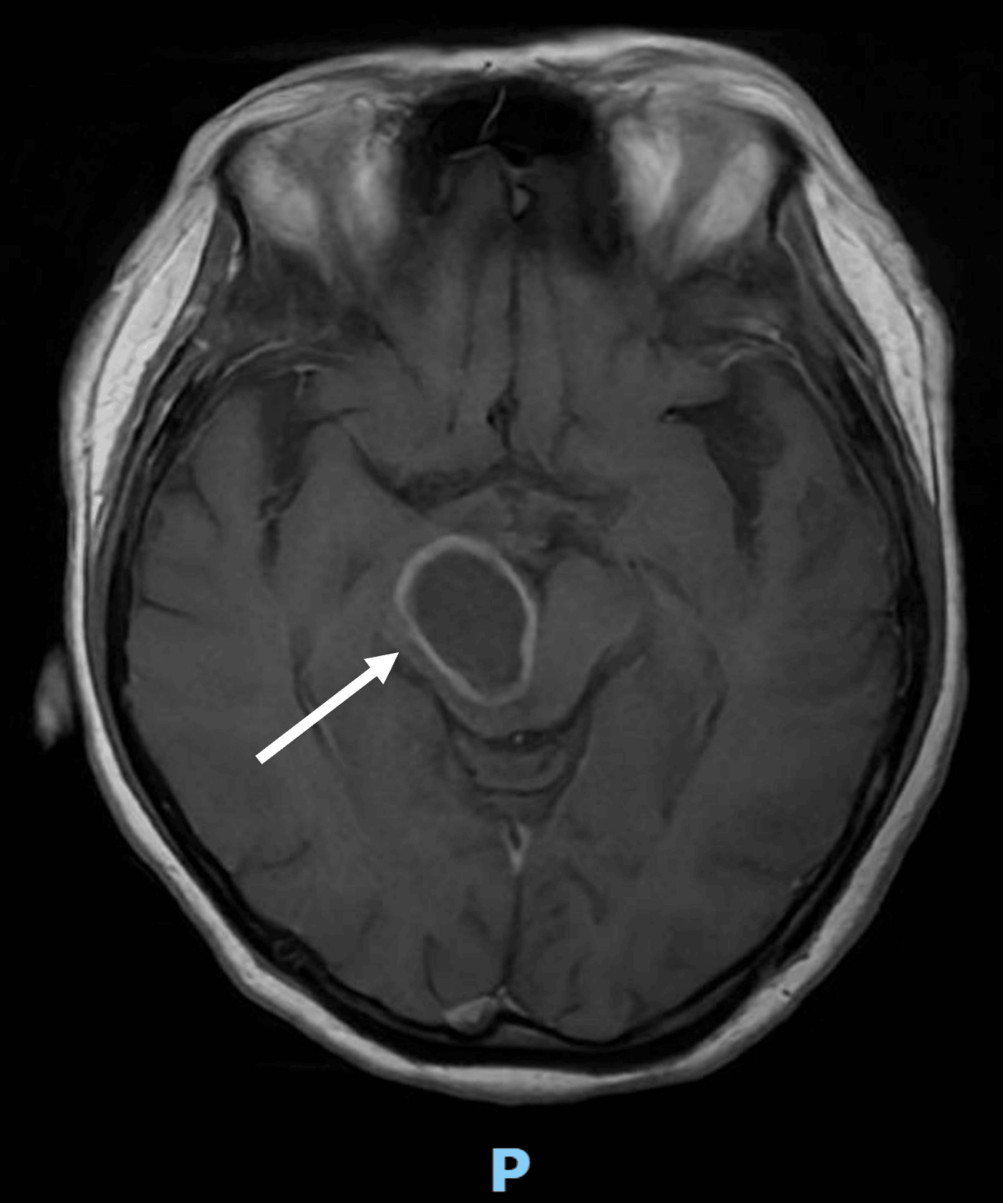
MRI Brain demonstrating interval increase in size and surrounding oedema of the known right midbrain/cerebral peduncle abscess (arrow)
